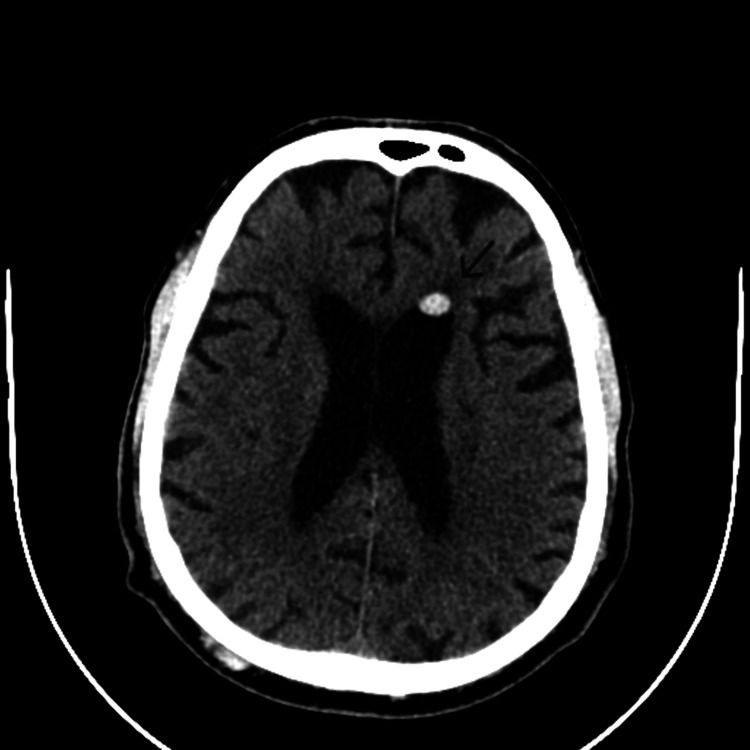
Follow-up non-contrast-enhanced brain CT showing new hyperdense focus in the frontal horn of the same ventricle (arrow)
